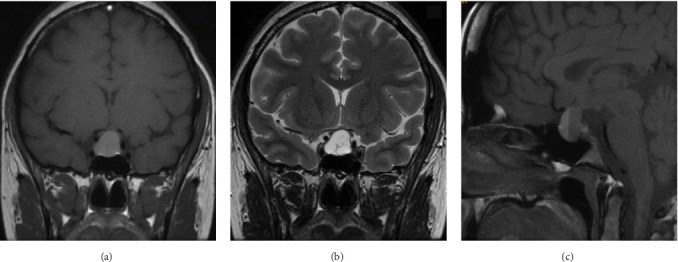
MRI without contrast. (A) T1 coronal view, (B) T2 and (C) T1 sagittal view. An increase in the size of the prolactinoma is observed, measuring 17 × 21 × 13 mm, compressing the chiasm. Presents fluid level and is hyperintense on T1 compatible with bleeding.
